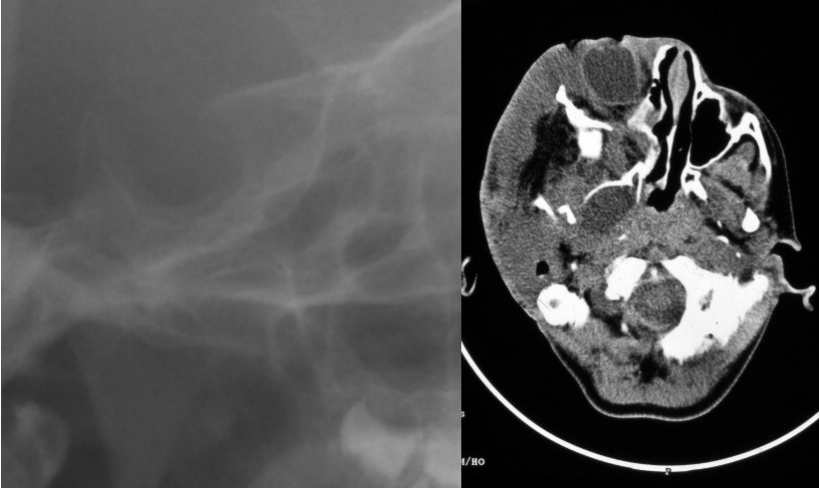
Left side: Cropped image of lateral cephalogram in a 9-year-old male patient with hemifacial PNF: enlarged sella turcica. Right side: Axial section image of computed tomography shows extremely wide foramen ovale on the side of facial PNF.
